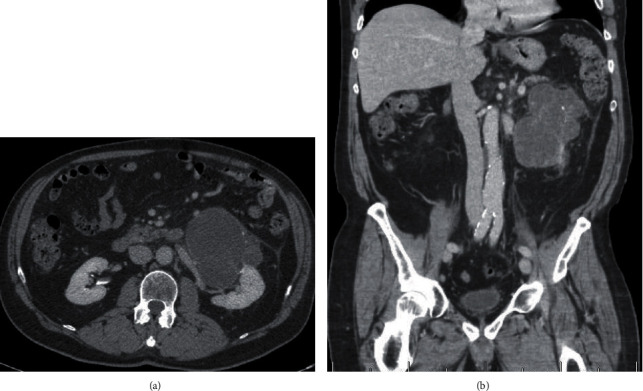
CT imaging after nivolumab/ipilimumab treatment: (a) axial imaging demonstrating significant decrease in size of the renal mass; (b) coronal imaging demonstrating resolution of the bulky retroperitoneal lymphadenopathy.
